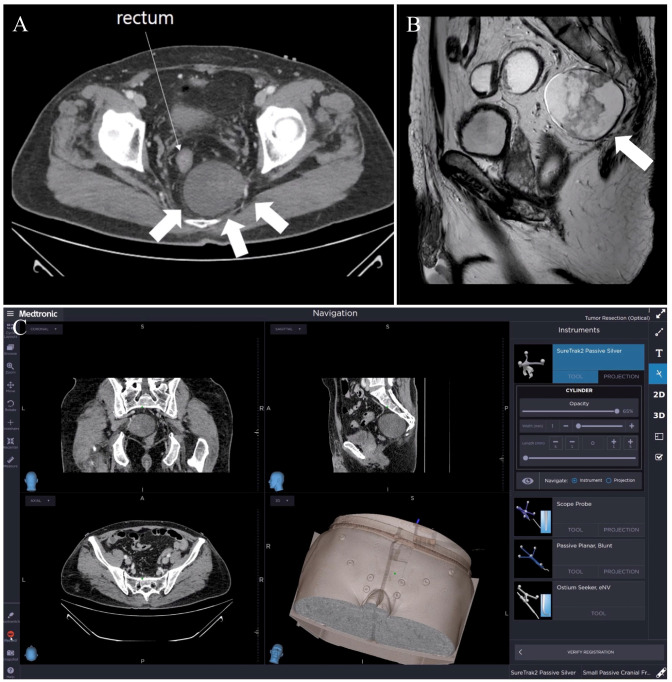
CT scan showing the retro-rectal tumor (thick arrow): (A) axial view and (B) sagittal view. (C) Multiplanar real-time view on the navigation software interface of the dissection above the tumor. The blue dot in each quadrant shows the instrument’s tip position for each plane.
